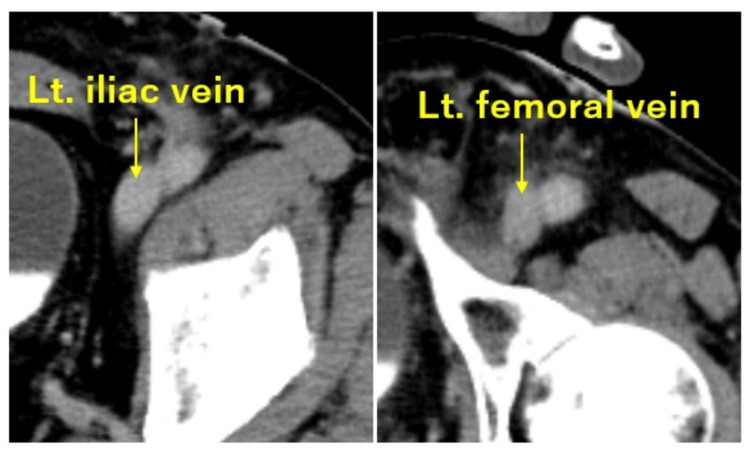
Postoperative computed tomography findings. No thrombus was detected in the iliac or femoral veins. Lt: left.
